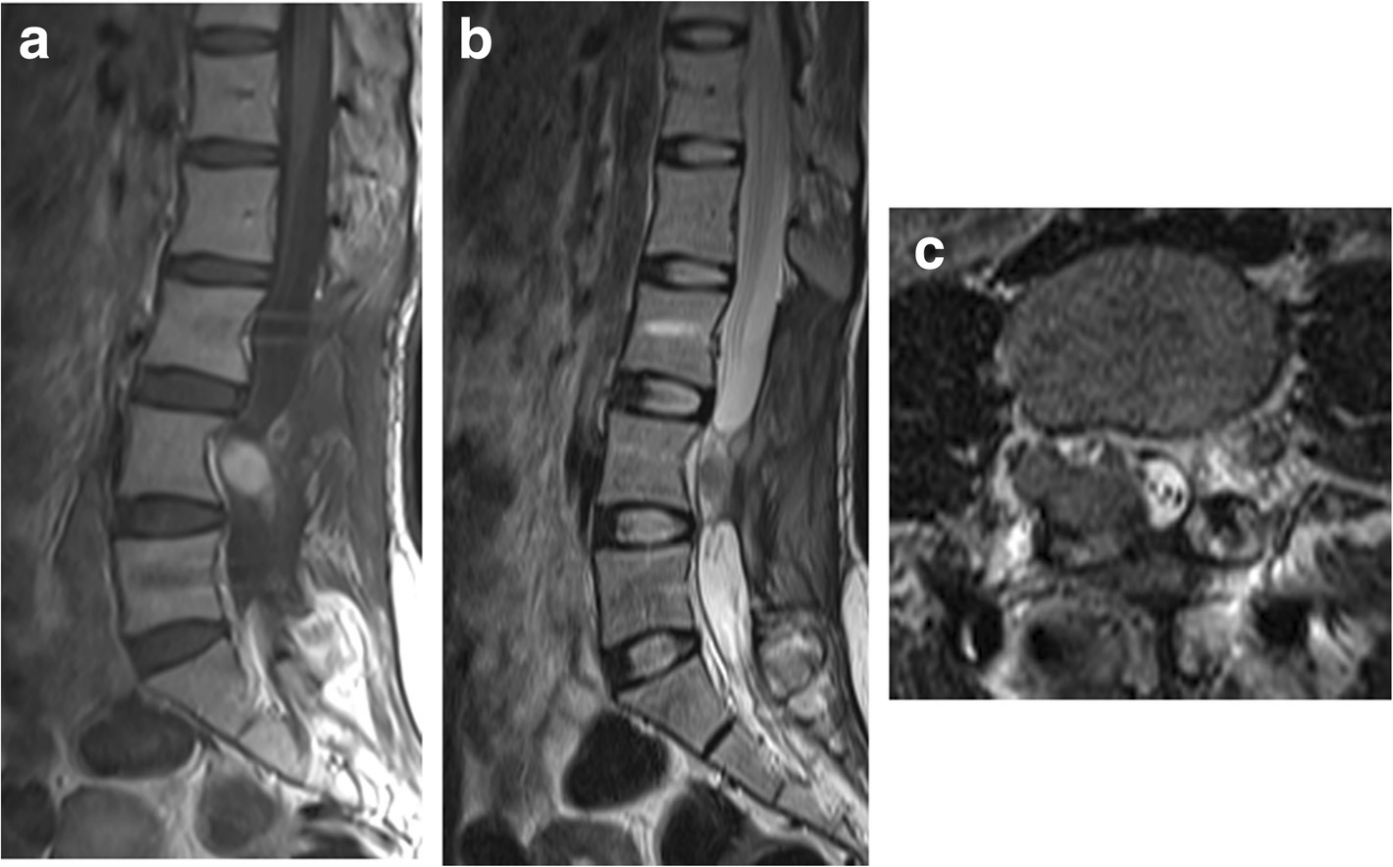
MRI images 5 months after the surgery. Sagittal T1WI (a), T2WI (b), and axial T2-WI (c) find a soft tissue mass at the L4 level of spinal canal, confirming tumor recurrence
